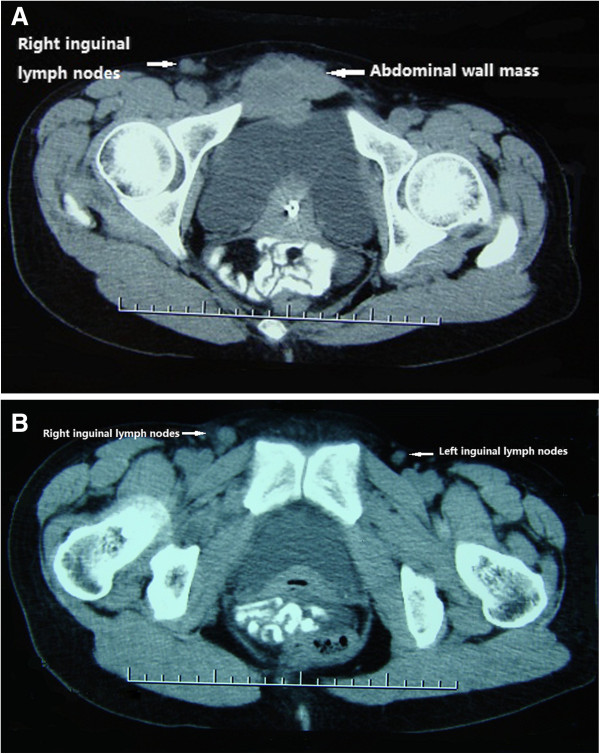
Computed tomography scans. The abdominal wall mass had invaded the bladder (A) and the inguinal lymph nodes (A, B).
